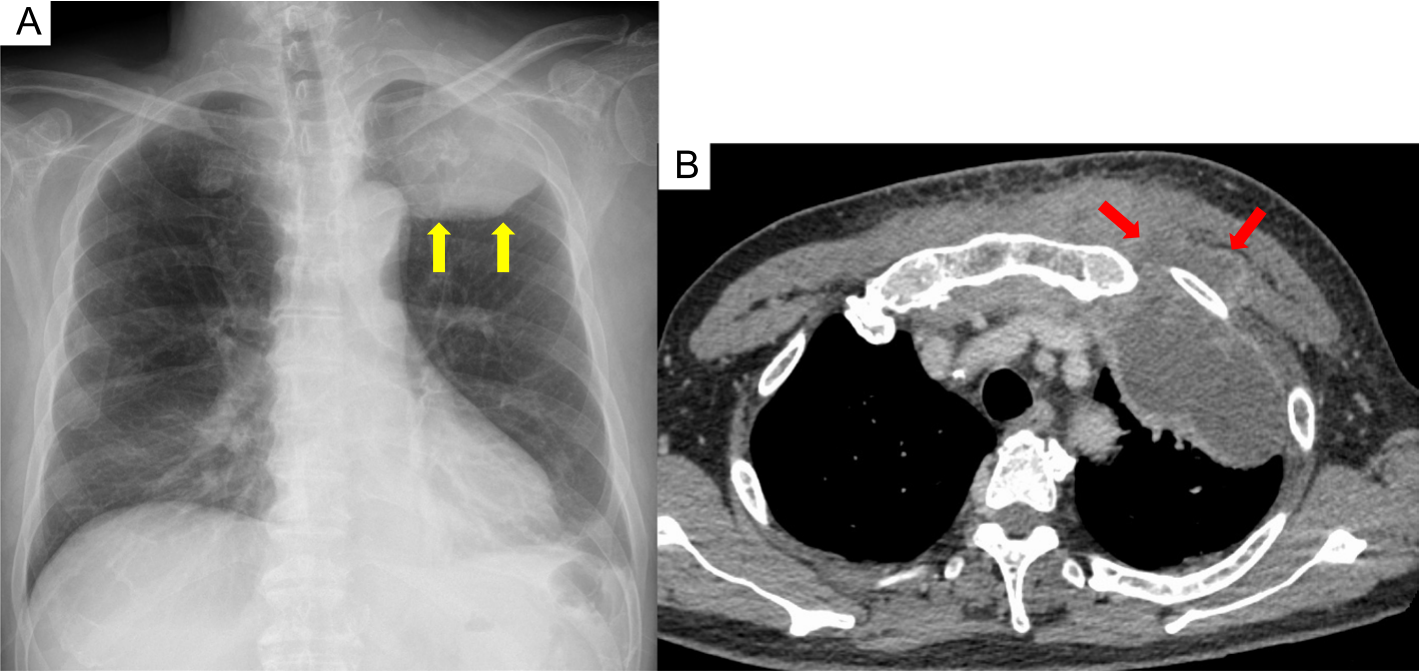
Chest radiography and contrast-enhanced chest computed tomography scan acquired on admission. a Chest radiography reveals an infiltrative shadow in the left upper lung field. b Contrast-enhanced computed tomography reveals fluid accumulation with marginal contrast enhancement around the sternocostal joint of the left first rib, extending subcutaneously
